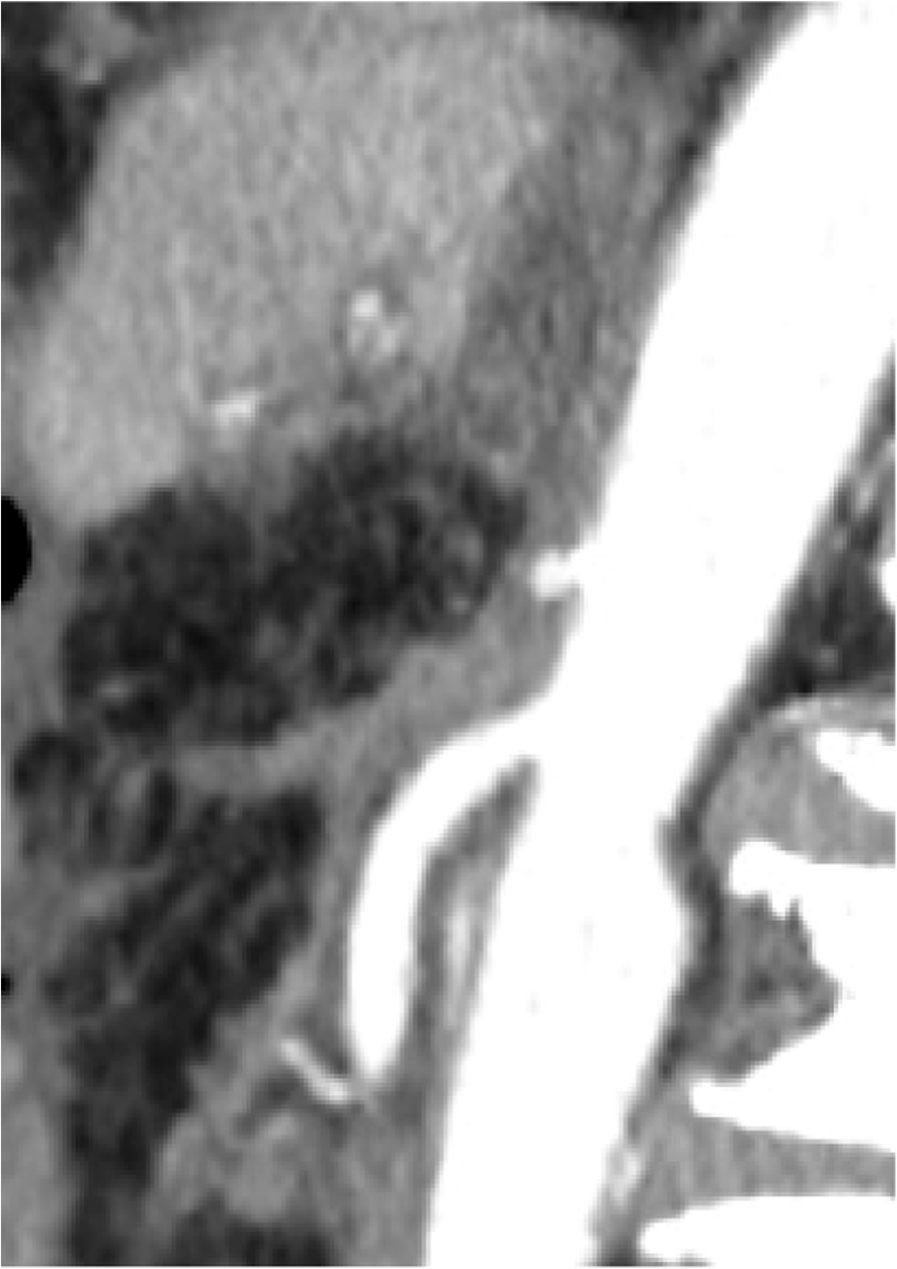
Contrast-enhanced multidetector CT at POD 20 showing occlusion of the celiac artery
